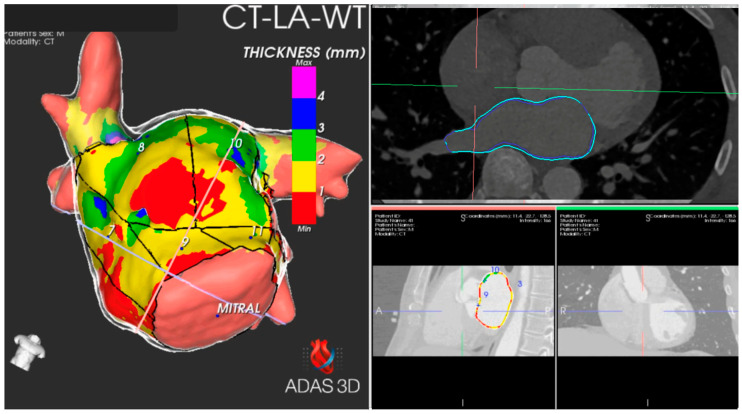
Software reconstruction using multidetector computed tomography images and analysis using ADAS 3D™ software generated a 3D map of the atrial wall thickness. Thickness colour map: thickness <1 mm, red; 1–2 mm, yellow; 2–3 mm, green; 3–4 mm, blue; >4 mm, purple.
